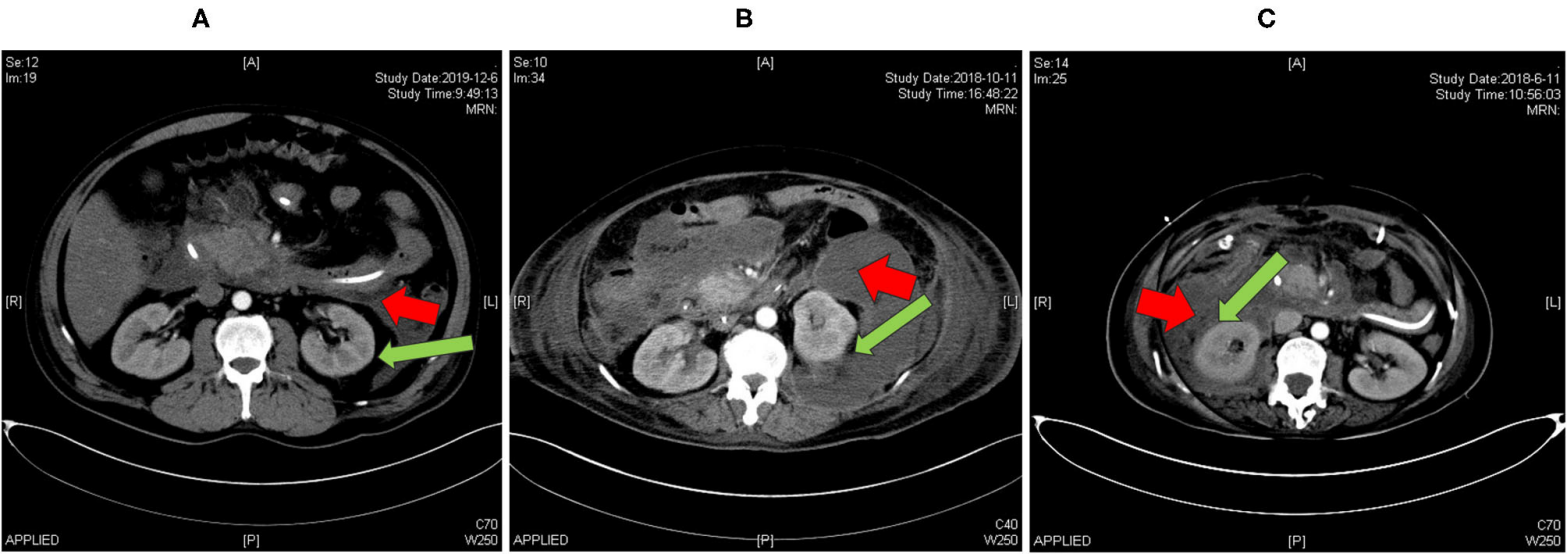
Abdominal CT imaging (arterial phase) of patients with AP included in our study. (A) A patient admitted to hospital in December 2019 with no involvement of renal capsule (RRG II); (B) a patient admitted to hospital in October 2018 with partial involvement of renal capsule (RRG III); (C) a patient admitted to hospital in June 2018 with full involvement of renal capsule (RRG III). Red arrow: pancreatic collections, green arrow: renal capsule. RRG, renal rim grade.
